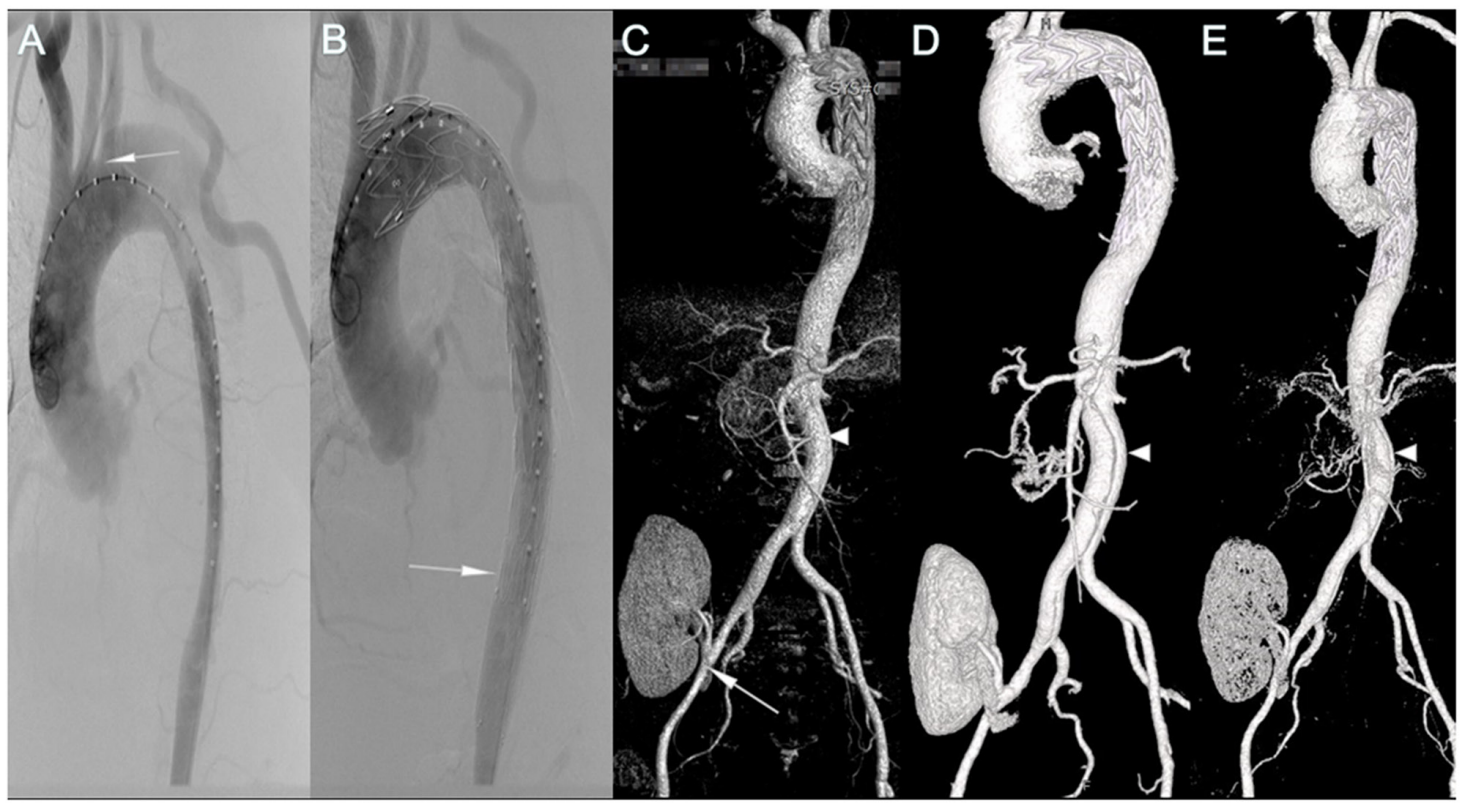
Pre-, Intra-, Post-operative imaging of patient 1 (A) Aortography showed that the true lumen was compressed at the primary entry tear located at the proximal descending aorta (arrow), 5 mm to the LSA. (B) Aortography after stent deployment showed successful endovascular repair of the dissection without endoleak. The bare stent was deployed at the distal descending aorta (arrow). The lumen was expanded by the stent. (C-E) The 1st, 6th, 24th month postoperative CTA showed that none of endoleak, malperfusion of renal graft, or stenosis of renal artery was occurred (arrow), but retrograde flow from distal tear site was still existed (triangle).
